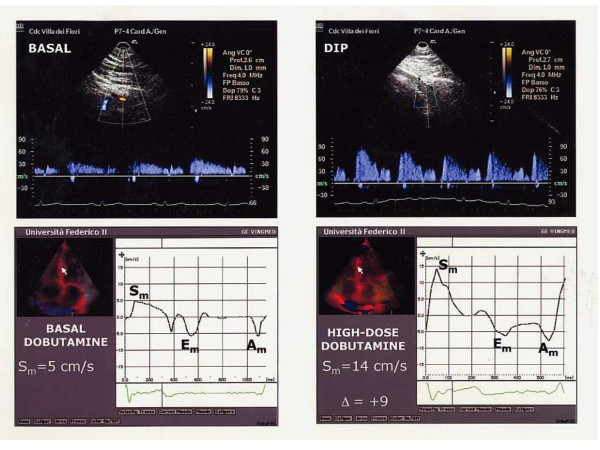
CFR and S_m _peak velocity of middle septum at high-dose dobutamine in a patient with SPECT derived normal perfusion. The upper panels show coronary artery flow velocity in the LAD at baseline and with a normal increase with dipyridamole (DIP). In the lower panels, myocardial systolic velocity (S_m_) shows a normal increase at high-dose dobutamine.
